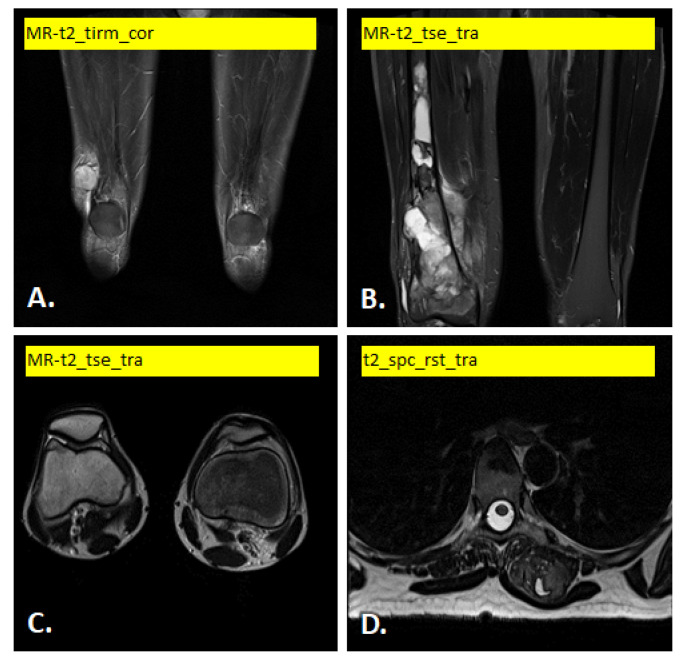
Example of T2-weighted MRI scan variants: (A) soft tissue tumor in lower end of right vastus lateralis muscle, (B) bone tumor in lower end of right femur, (C) soft tissue tumor in synovia of right knee joint and (D) soft tissue tumor in left erector spine muscle.
